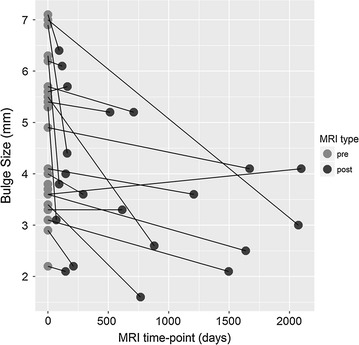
IVD herniation bulge measurements on MRI at pre-treatment compared to last post-treatment MRI shown by number of days after treatment. Lower values at the post-treatment time point indicates reduction in disc bulge size
